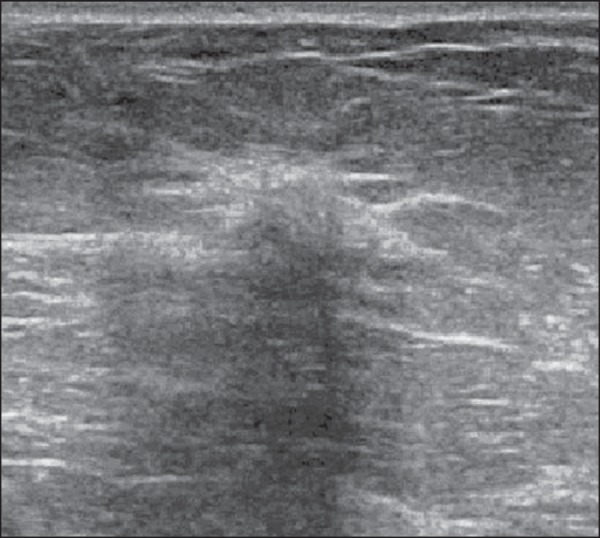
Intraductal papiloma*. Ultrasonography showing
